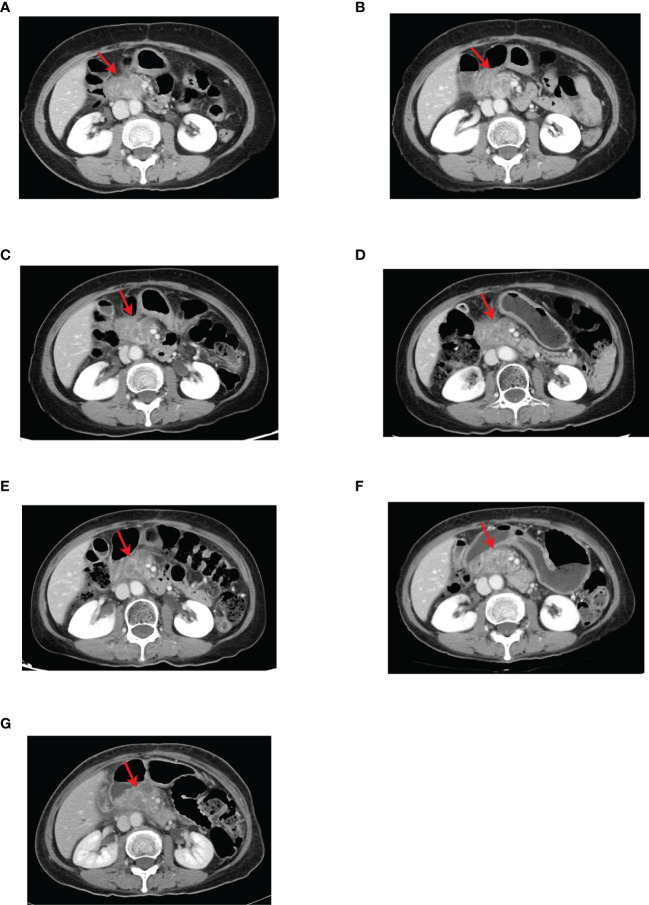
CT images of the pancreatic head lesion in the second-line course with red arrows indicating the location of the pancreatic head lesion. (A) Before anlotinib-targeted therapy combined with TS-1, the tumor size was about 2.4 cm × 4.3 cm. (B) CT after three cycles of anlotinib targeted therapy combined with TS-1 showed that the pancreatic head tumor was reduced to approximate 2.5 cm × 3.3 cm. (C) CT after eight cycles of anlotinib targeted therapy combined with TS-1 showed that the pancreatic head tumor was slightly enlarged compared with that after three cycles of treatment, about 4.2 cm × 3.1 cm. (D) CT showed no significant change in the size of the pancreatic head tumor after adjusting the anlotinib dose for two cycles. (E) After three cycles and (F) five cycles, CT showed that the pancreatic head tumor was slightly larger than the previous, about 4.3 cm × 3.1 cm. (G) CT image after adjusting the dose of anlotinib for six cycles showed no significant change in the size of the pancreatic head tumor compared with the previous, and the efficacy was evaluated as SD.
